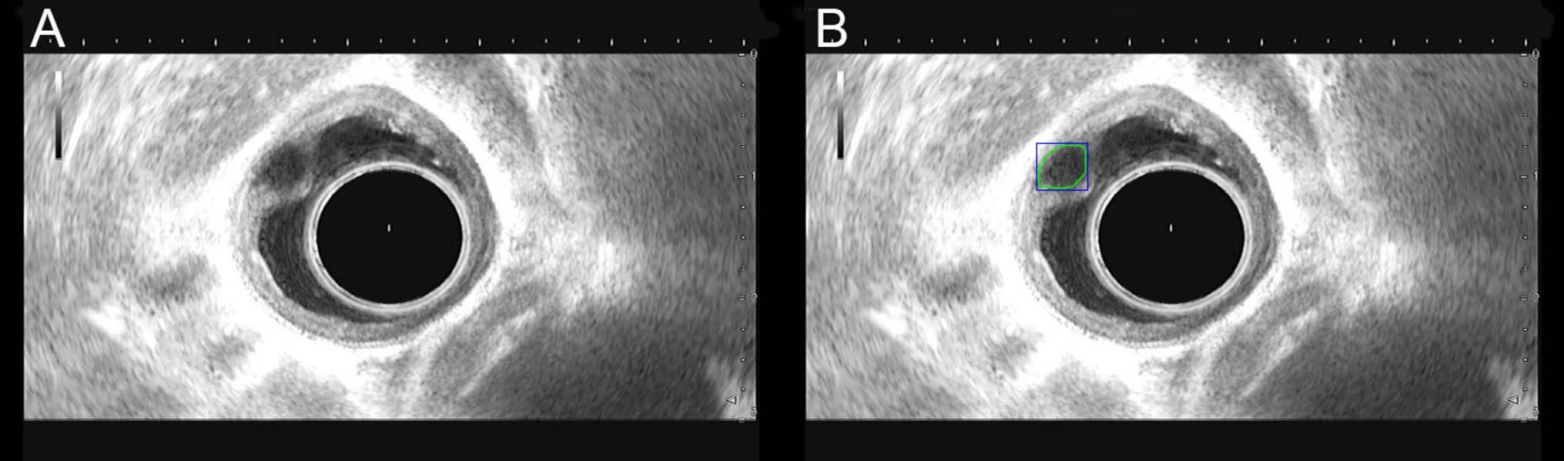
Example of test set image labeling. A Representative image of esophageal SELs. B AI model recognition image. The green frame indicates the diagnosis by the AI system. *SEL* subepithelial lesion, *EUS* endoscopic ultrasound
